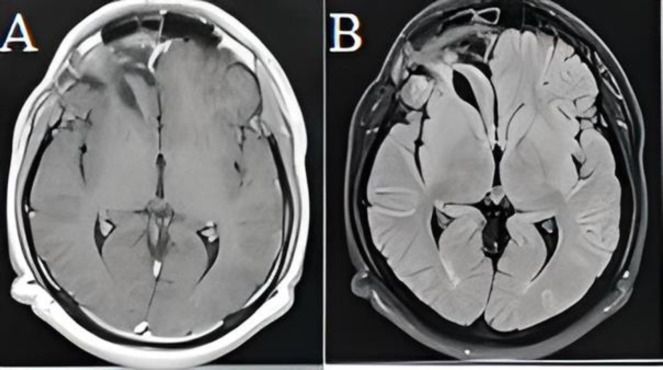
Axial sections of a brain MRI on T1-WI (A) and T2-DWI (B) showing a complete regression of the lesion
